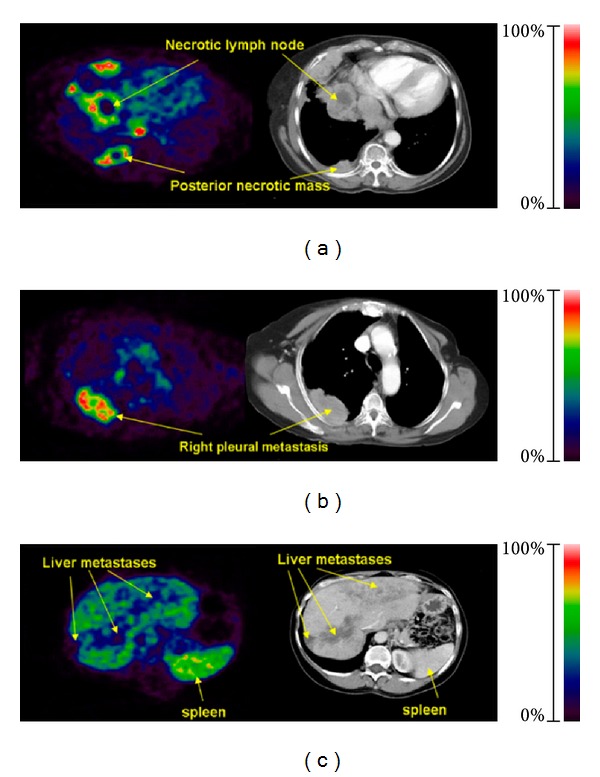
[^18^F]Fluciclatide PET: (a) patient with lung and pleural metastases. (b) Intralesion heterogeneity of uptake within pleural metastasis in PET image, which was not demonstrated as necrosis on corresponding CT section. (c) Liver metastases imaged as hypointense lesions because of high background signal (high uptake in spleen is possibly due to blood pooling) (with permission from Kenny et al. [18]).
